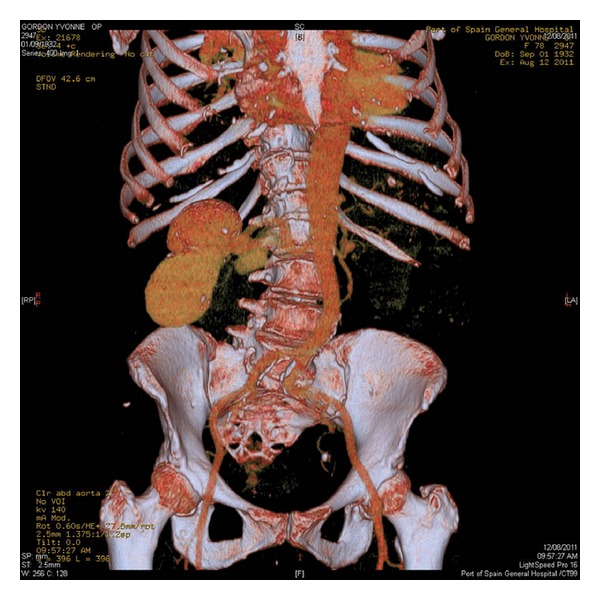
CT angiogram reconstruction showing a complex bilobular right renal artery aneurysm of approximately 10 cm wider diameter and a smaller aneurysm of 3 cm involving the distal renal artery and extending into the renal parenchyma.
